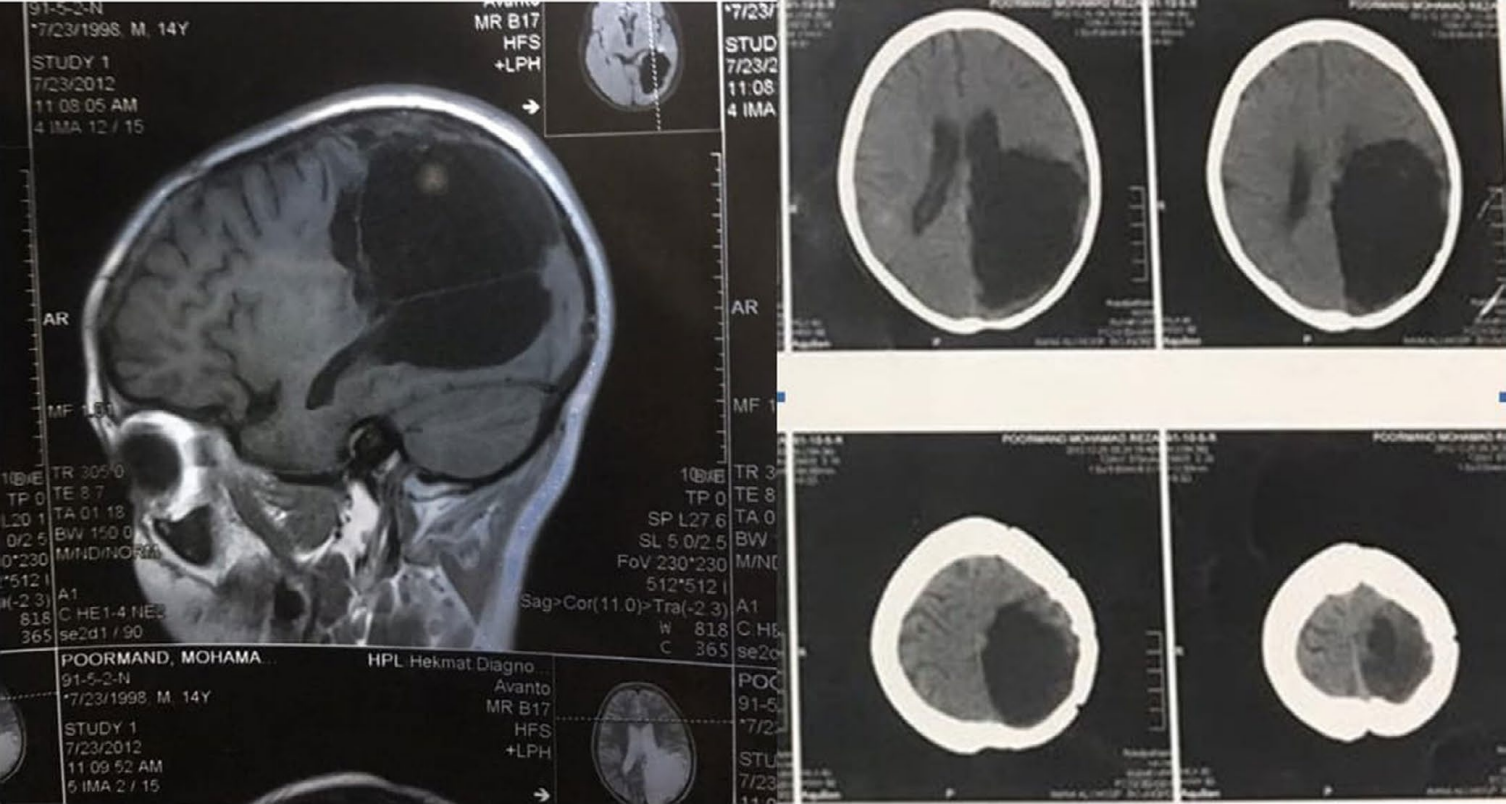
Postoperation follow‐up CT (right) and MRI (left) images shows post‐op changes with surrounding edema that reveals extraction of cystic lesions
